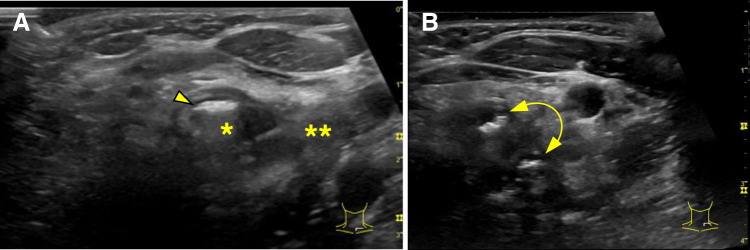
US findings. (A) US demonstrating a cystic mass consisting of 2 cavities. The cephalad cyst (asterisk) contains internal air (arrowhead) and has a wall structure resembling those of the gastrointestinal tract. The caudal mass (double asterisk) is filled with highly viscous fluid. (B) Air passage between the cyst and the normal esophagus (bidirectional arrow). US, ultrasonography
